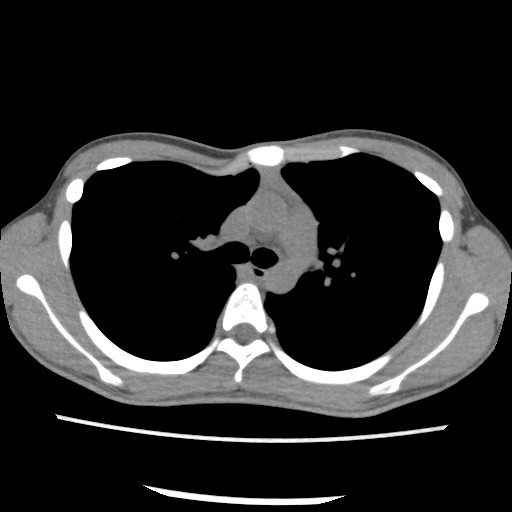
Chest computed tomographic scan showing asymmetric depression of chest wall.
